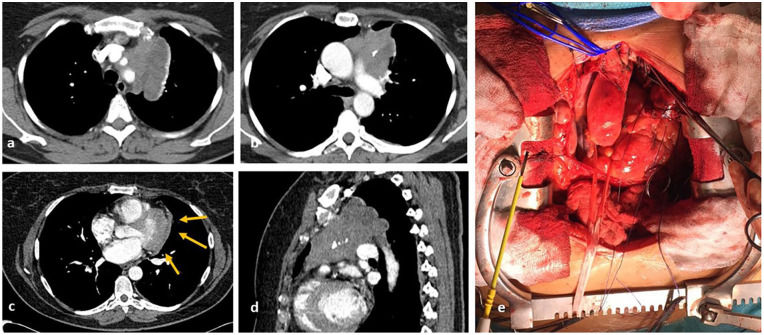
Preoperative computed tomography shows involvement of ascending aorta and main pulmonary artery, with possible extension surrounding the proximal descending aorta ([a], [b], and [d]). The mass also involves the pericardium around the left ventricle (arrows [c]). Complete macroscopic resection was achieved (d).
